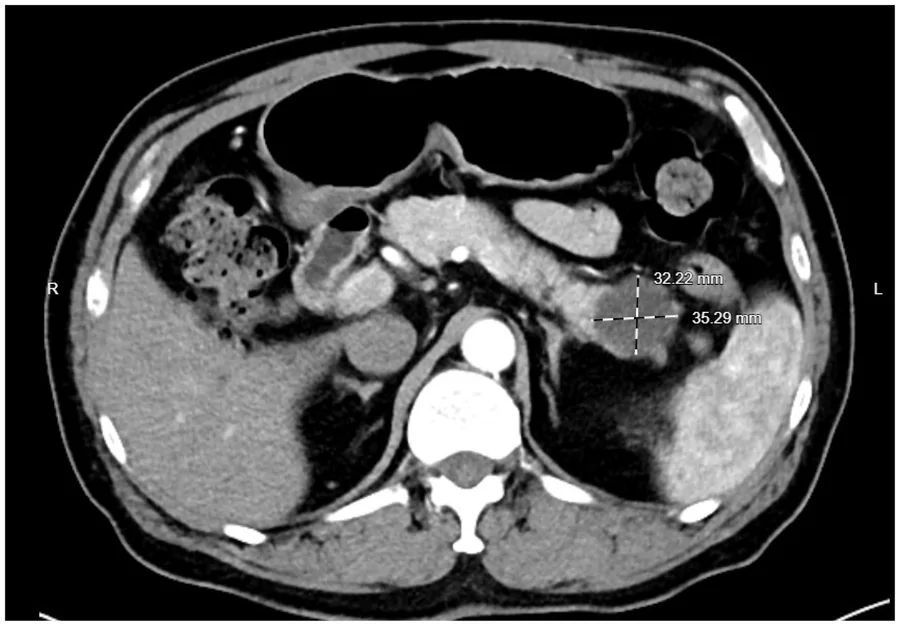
Preoperative contrast-enhanced abdominal computed tomography. Arterial-phase contrast-enhanced abdominal computed tomography obtained on February 15, 2025, showing a cystic lesion in the pancreatic tail measuring approximately 35.3 × 32.2 mm in the axial plane.
